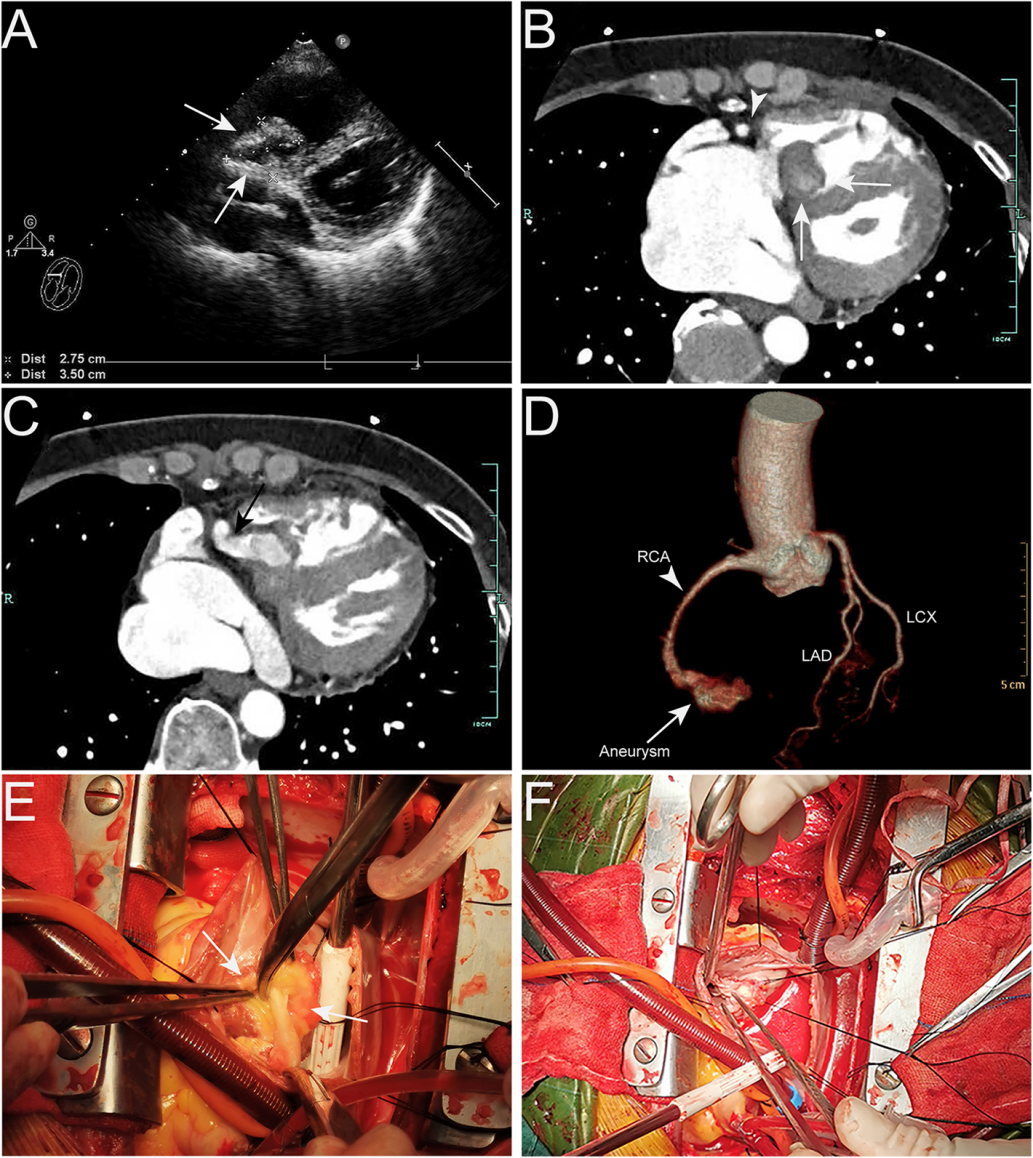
(A) TEE revealed a 3.5 × 2.7 cm cystic mass (white arrows) with hyperechoic external capsule locating below the annulus of posterior tricuspid leaflet; (B, C) Coronary CTA revealed an irregular mass (white arrows) with internal contrast enhancement and thick external capsule locating below the tricuspid valve. A feeding vessel (black arrow) originating from RCA (white arrow head) was identified; (D) 3D reconstruction of coronary arteries confirmed that the mass was supplied by RCA and left coronary artery disease was eliminated; (E) After detaching the posterior leaflet from the tricuspid annulus, the mass (white arrows) was detected with collagen-like degenerative fibrous coating; (F) The RCA aneurysm was resected and the posterior leaflet was repaired and reattached to the annulus by continuous suture with 5–0 prolene. TEE, transthoracic echocardiography; CTA, computed tomographic angiography; RCA, right coronary artery
